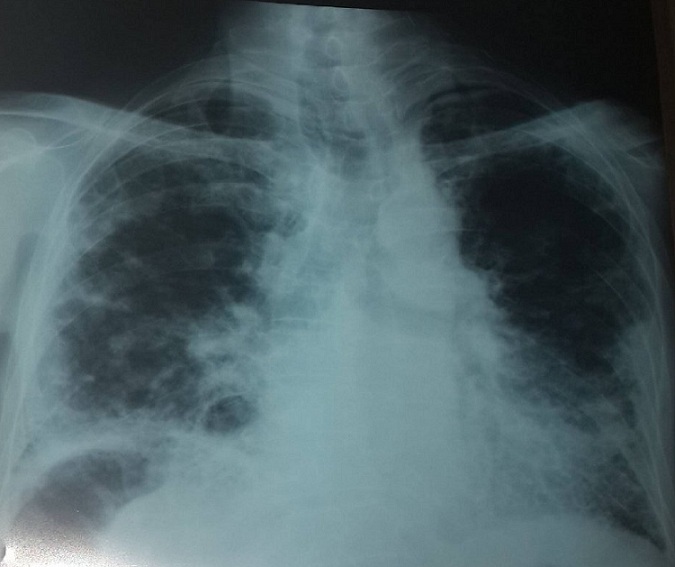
Radiographie thoracique face à l’admission: opacités réticulomicronodulaires diffuses à répartition périphérique et basale
